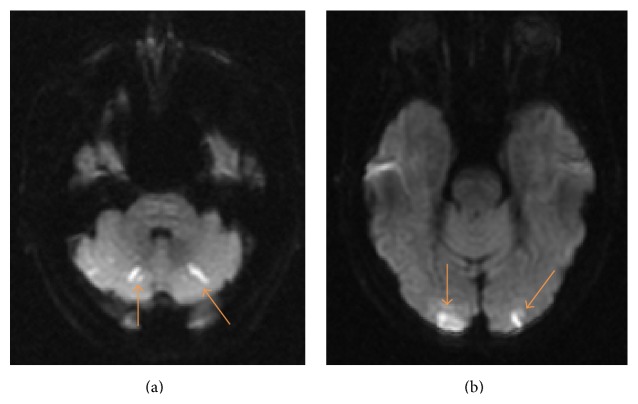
Acute ischemic infarct, MRI brain DWI sequences. (a) Bilateral cerebellum. (b) Bilateral occipital lobes.
